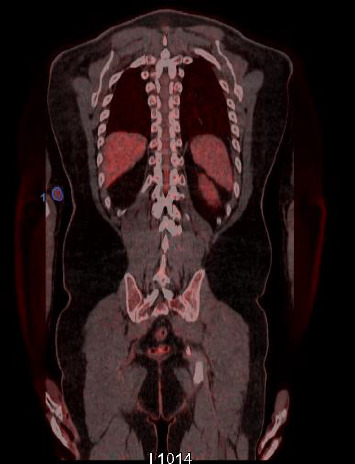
PET exam. Tracer distribution images (335 Mbq 18F-FDG) detected pathological uptake only at the level of the right epitrochlear lymph node region.
